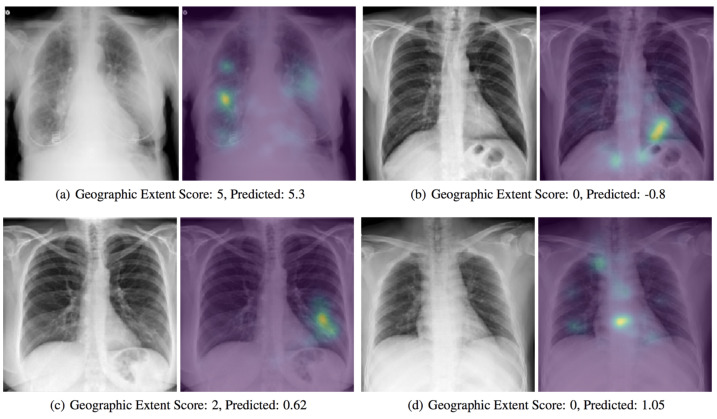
A presentation of a predicted severity scores for COVID-19 chest X-ray scans while using the DenseNet model [48].
